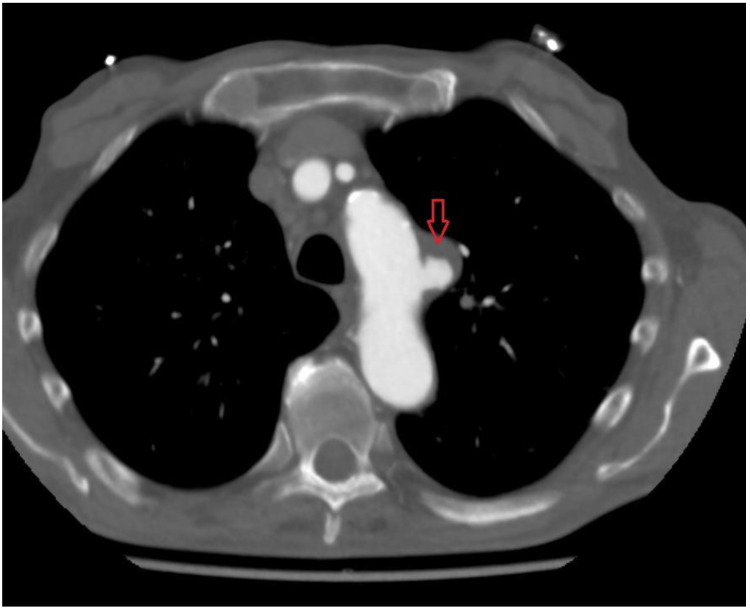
Preprocedural CT scan; red arrow shows PAU.
